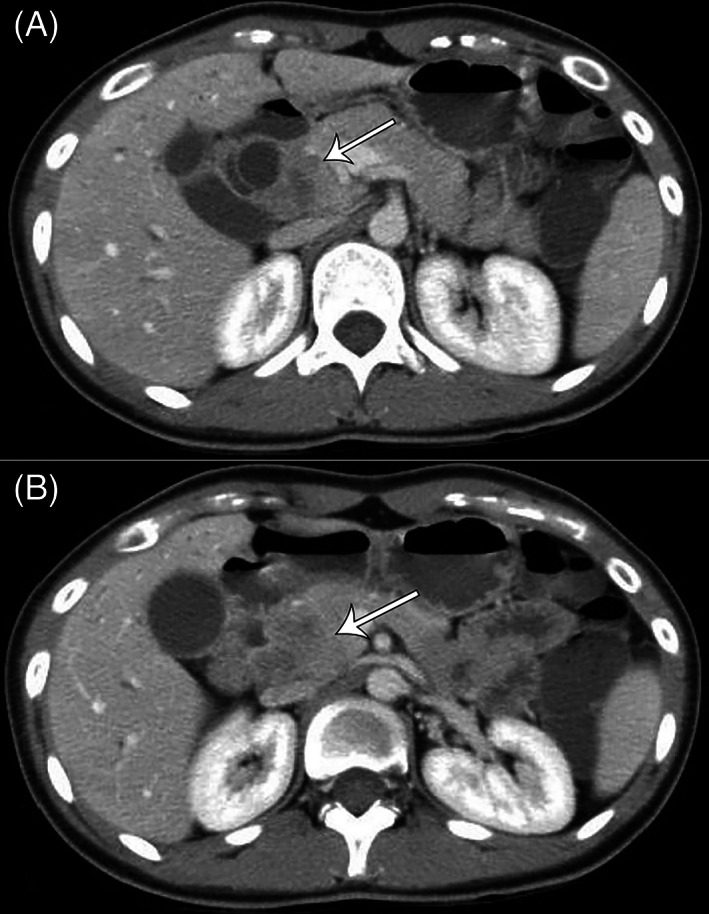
Spiral Computerized Tomography (CT) scan of the abdomen with intravascular (IV) and oral contrast (A, B) showcases a bulged head of pancreas with heterogeneous enhancement, hypodense foci measuring 15× 30 mm is seen in the corresponding area, in close proximity to hepatic vessels. Gallbladder, cystic and common bile ducts are dilated.
